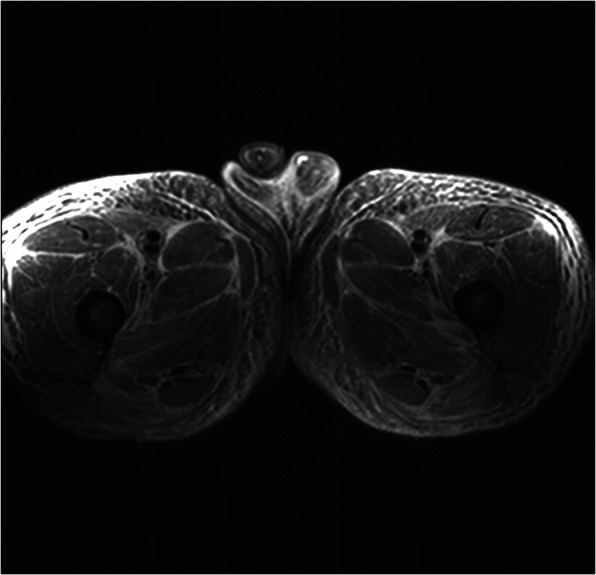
Magnetic resonance imaging (MRI) indicating myositis. Diffuse high intramuscular and extramuscular signals showing edema consistent with myositis (MRI short tau inversion recovery sequence, axial views)
